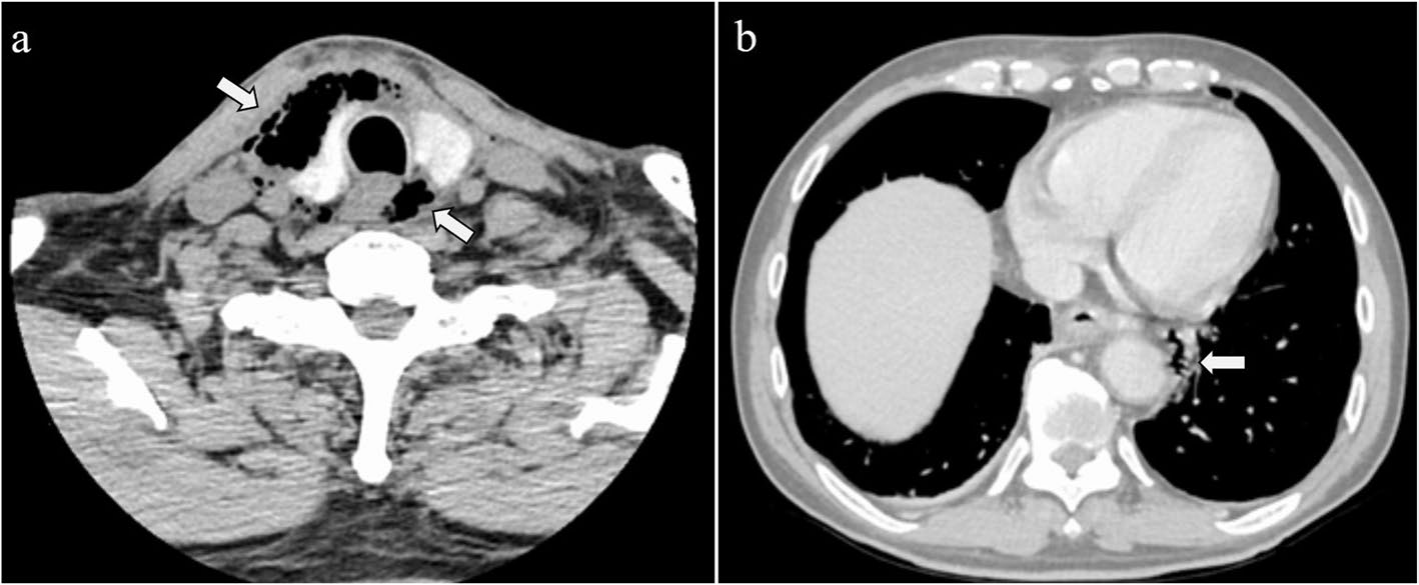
Cervicothoracic computed tomography (CT) image showing gas bubbles in the cervical spaces (a) and posterior mediastinum extending toward the esophagogastric junction (b). Arrows in a and b indicate gas bubbles
